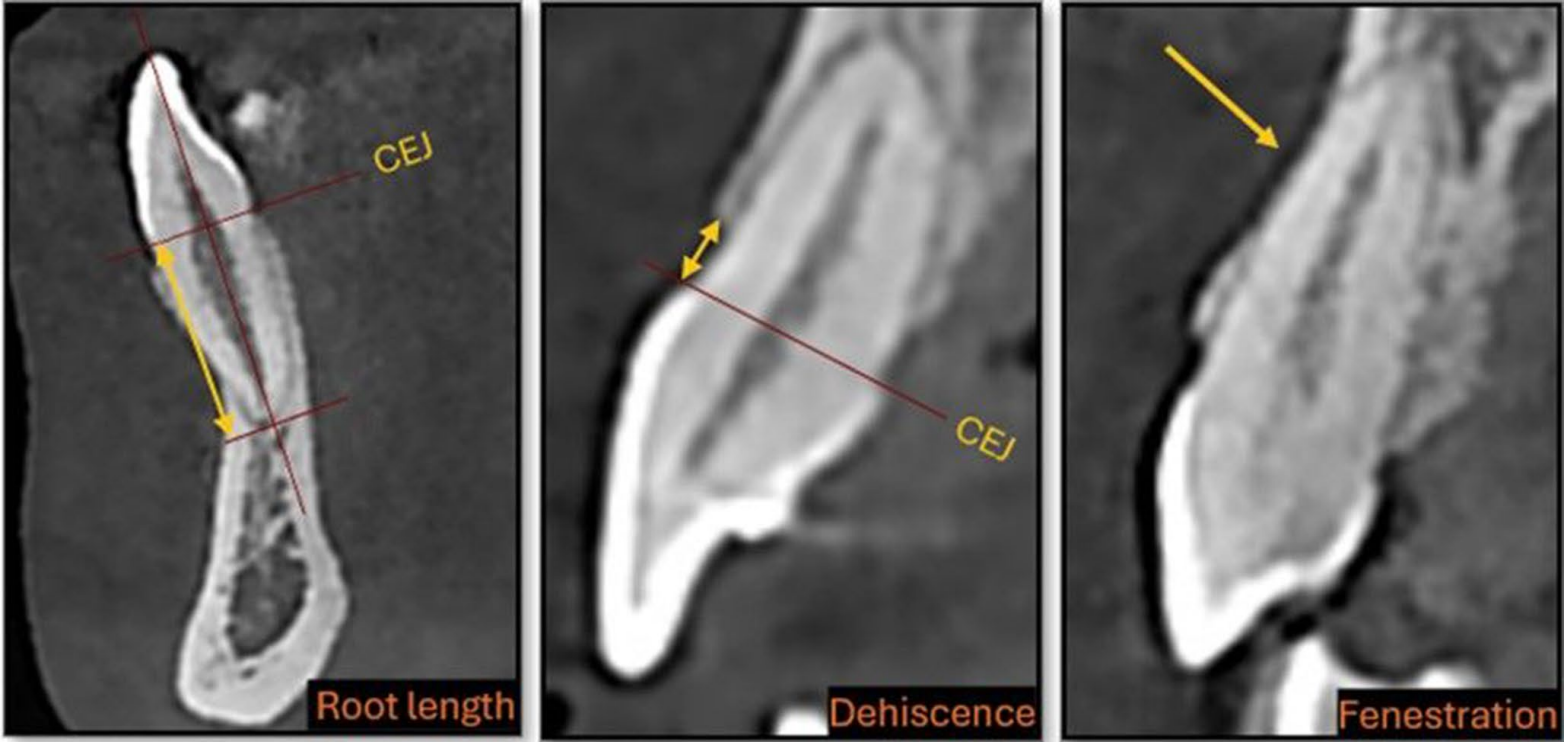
Assessment of root length and alveolar bone defects (dehiscence and fenestration) on CBCT images. *CEJ* cemento-enamel junction
